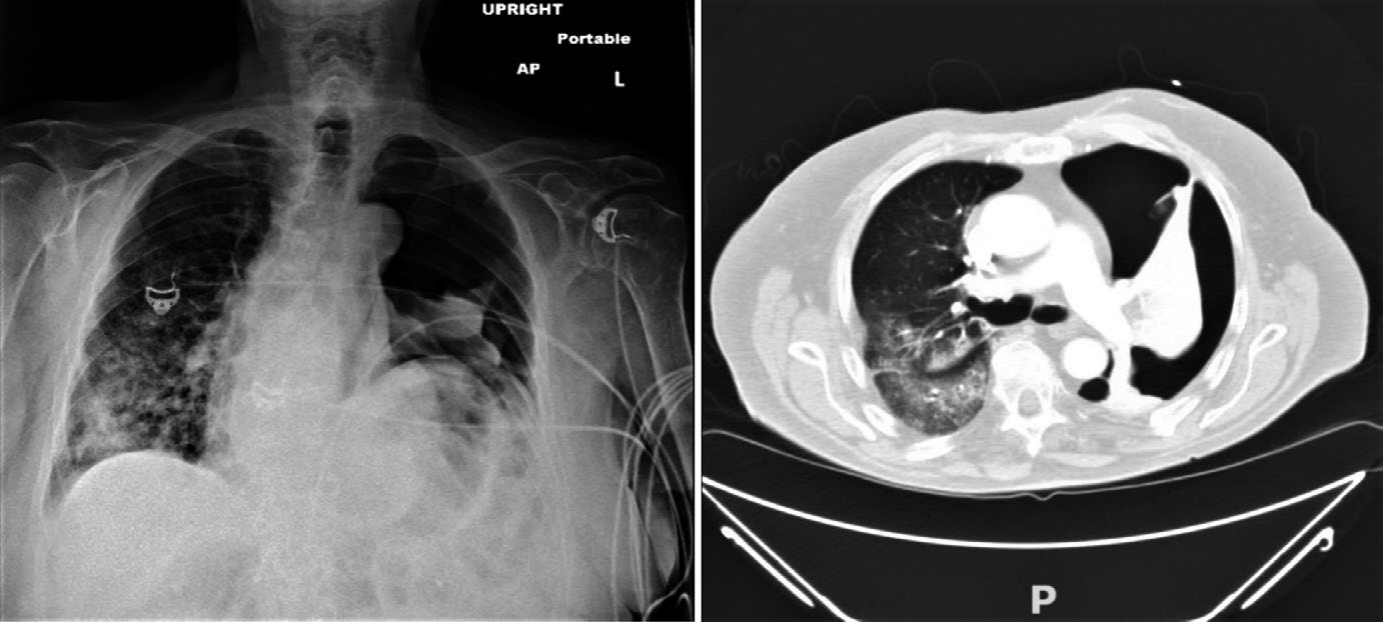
Panel A shows a large left pneumothorax; the left hemidiaphragm is elevated, which is a chronic finding. Panel B is a CT scan of the lung with IV contrast showing large left pneumothorax with lung collapse and showing no evidence of pulmonary embolism; there are ground‐glass opacities in the right lung consistent with known COVID‐19
